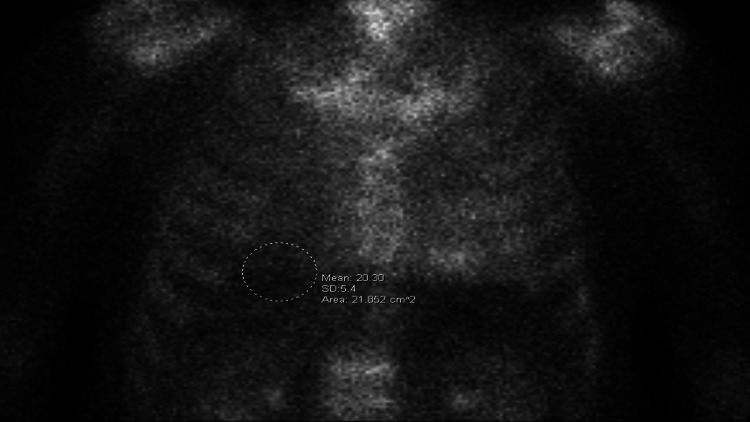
The patient’s technetium-99m pyrophosphate nuclear scintigraphy indicates a heart-to-contralateral lung ratio, calculated by comparing the tracer activity in the heart to that in the contralateral lung (dotted circle), of 1.6.
